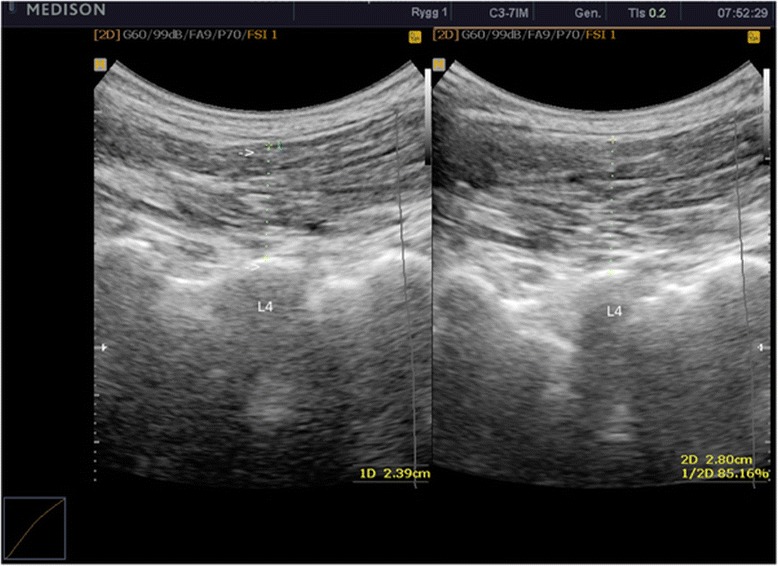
Ultrasound image of resting LMM (left image) contracted LMM (right image). Calipers placed on the apex of facet joint of L4, and on the interface between the thoracolumbar fascia and subcutaneous fat.
